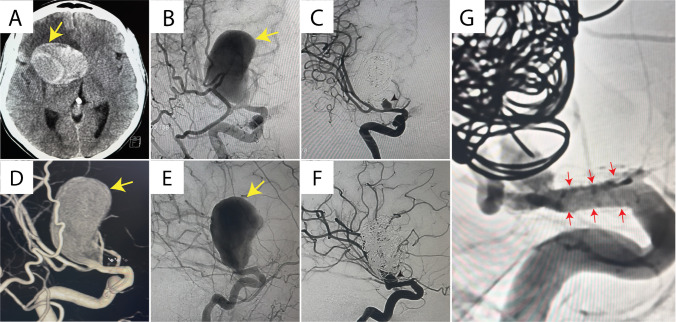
51-year-old Caucasian female with unruptured giant partially thrombosed right middle cerebral artery (MCA) aneurysm causing mass effect and presenting with syncope, treated with coil embolization and Surpass Elite flow diverter. A Axial non-contrast head computed tomography demonstrating partially thrombosed giant aneurysm causing mass effect (yellow arrow). B, E Anteroposterior (AP) and lateral angiographic views of right internal carotid artery (ICA) demonstrating filling of a giant proximal right M1 MCA aneurysm measuring 35 × 21 × 31 mm with a 5.6 mm neck (yellow arrow) with irregular contours and superior projection. D Rotational 3D angiogram demonstrating filling of the giant right MCA aneurysm. C, F Three-month follow-up AP and lateral angiographic views of right ICA after placement of coils and single Surpass Elite flow diverter (3.75 × 15 mm) extending from the ophthalmic segment of right ICA to the mid-M1 segment across the aneurysm neck, demonstrating complete dome obliteration with residual filling of the aneurysm neck (black arrowhead). G Magnified unsubtracted lateral projection angiogram of right ICA showing residual aneurysm neck and remodeling of the parent ICA with minimal intimal hyperplasia (red arrows)
